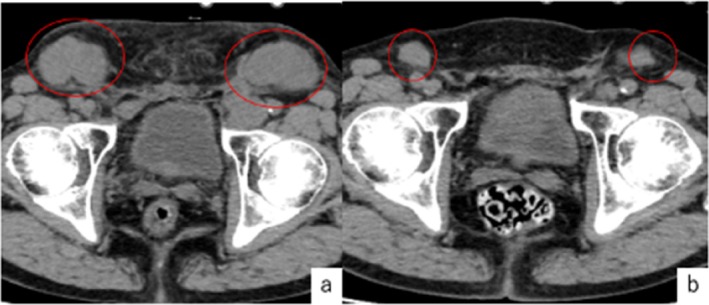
Radiological response of lymph node metastases to nivolumab on CT: Before treatment (a) and after treatment (b).
